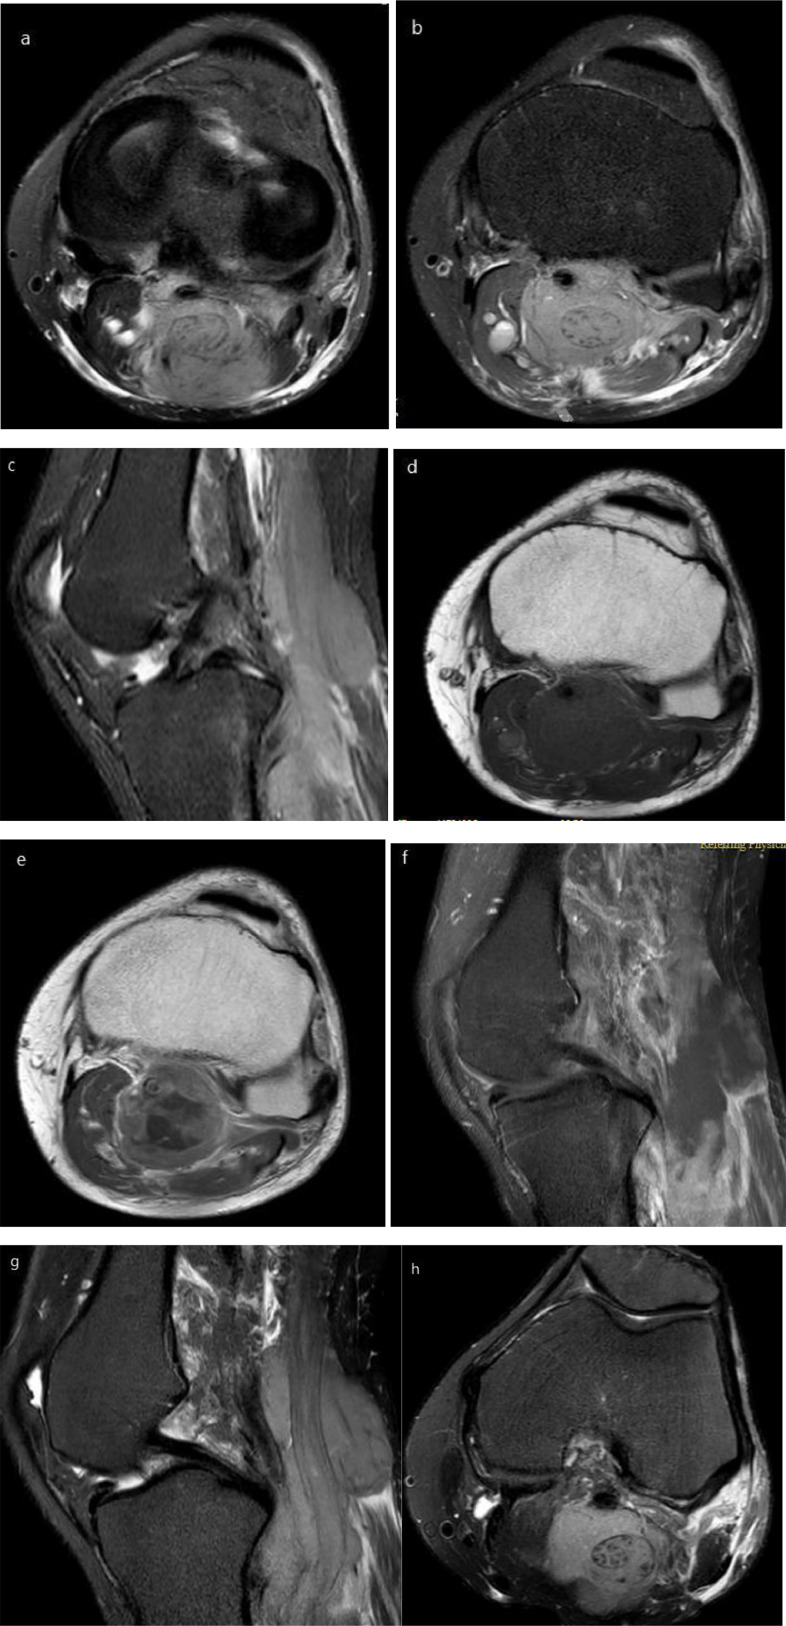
MRI of the left knee. A–H. (A) Axial T2; (B) Axial proton density fat-saturated; (C) sagittal STIR; (D) axial T1-weighted; (E) axial postcontrast T1-weighted; (F) sagittal postcontrast T1-weighted fat-saturated; (G) sagittal T2-weighted fat-saturated and (H) axial T2-weighted fat-saturated. Selected MRI images demonstrated a large fusiform mass with heterogeneous high signal intensity in T2-weighted and intermediate signal intensity in T1-weighted along the course of tibial nerve, which is enlarged and infiltrated. Heterogeneous predominantly peripheral enhancement is seen in postcontrast T1-weighted images.
